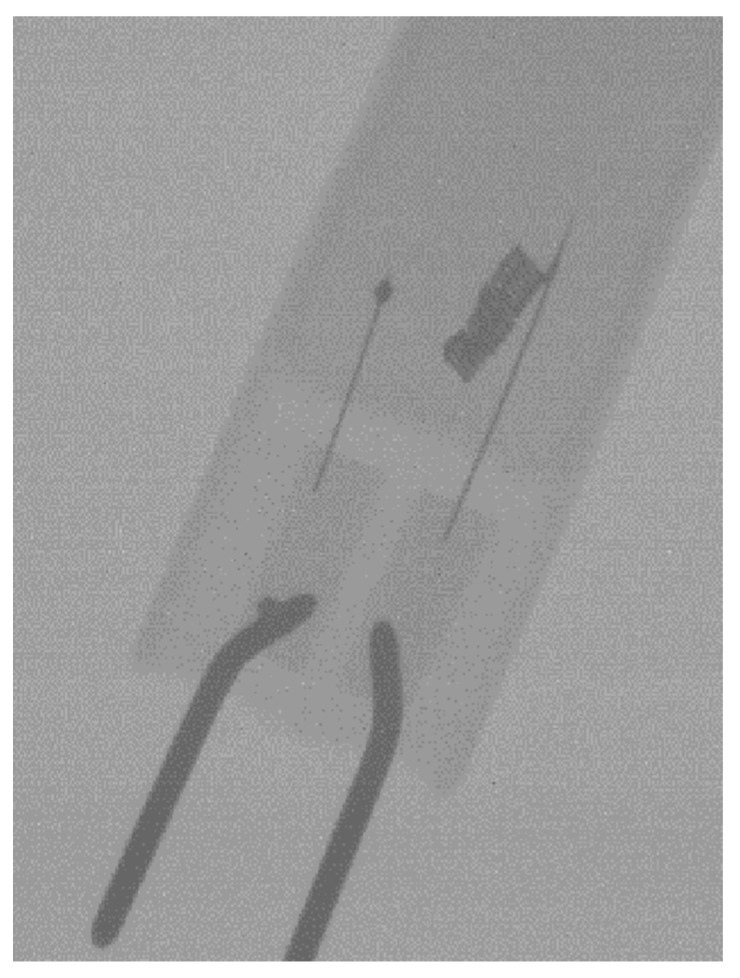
Halogen lamp radiography on the Remote RadEye HR (70 kVp 20 mAs).
